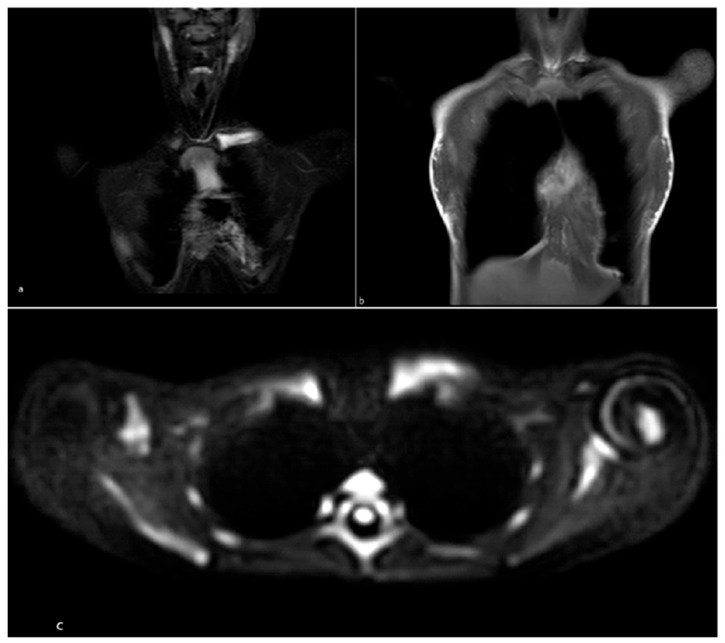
Patient 3 with diagnosis of CNO. (a) Whole-body MRI, T2 STIR coronal: marked signal hyperintensity at the medial end of the left clavicle; (b) Coronal T1 image showing hypointensity at the same site; (c) DWIbs (Diffusion Weighted Imaging with background suppression).
